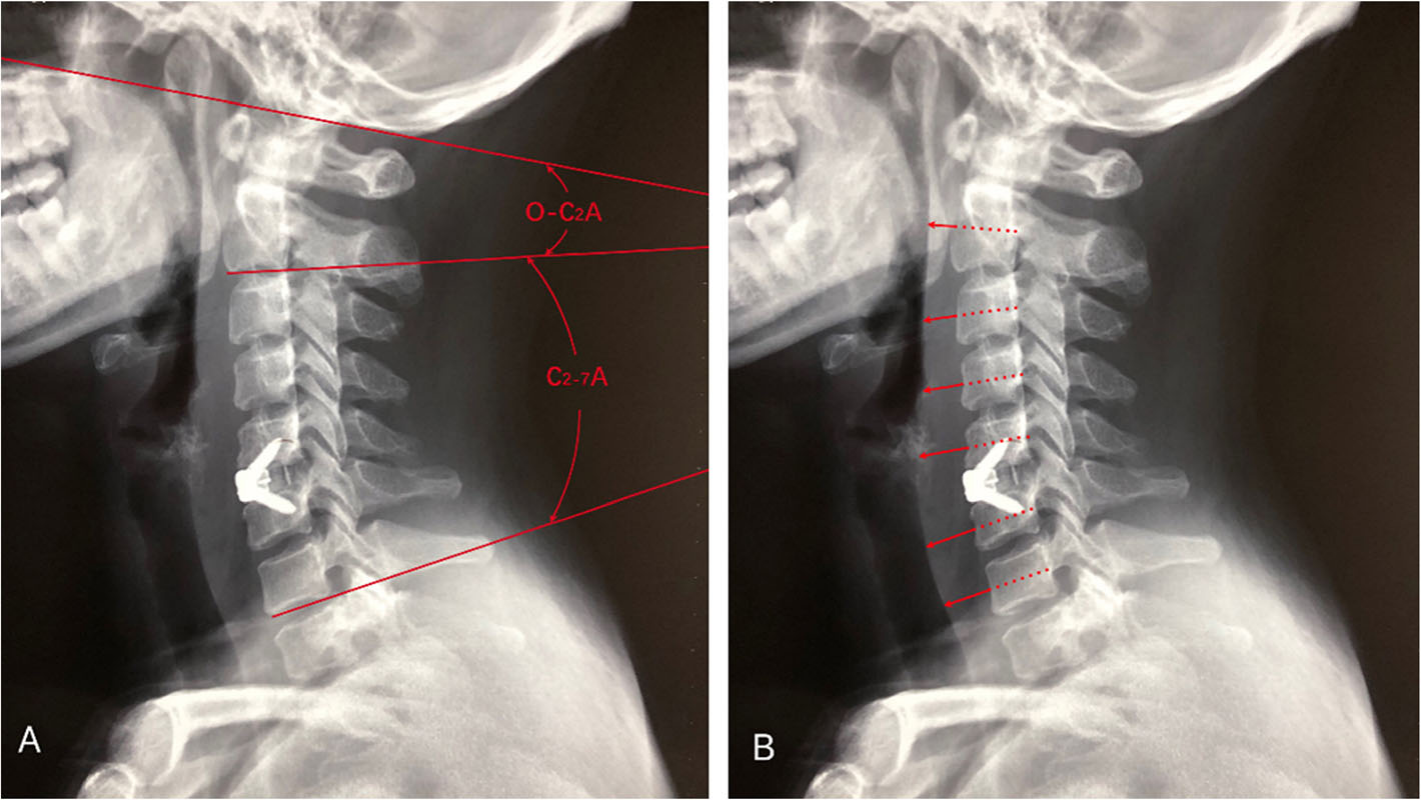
The measurement of O-C_2_A, C_2–7_A(a) and PSTT(b) on postoperative lateral X-rays. The angle between the inferior margin of the C_2_ vertebrae and the inferior margin of the C_7_ vertebrae was measured as the C_2–7_ angle while the angle between the McGregor’s line and the inferior margin of the C_2_ vertebrae was measured as the O-C2 angle perioperatively on plain lateral cervical radiographs (a). The PSTT was measured at the extended line of the AP diameter from C_2_ to C_7,_ and the AP diameter was measured between the center of the posterior and anterior cortex of each vertebral body. O-C_2_A: O-C_2_ angle, C_2–7_A: C_2–7_ angle, AP: Anteroposterior, PSTT: Prevertebral soft tissue thickness
